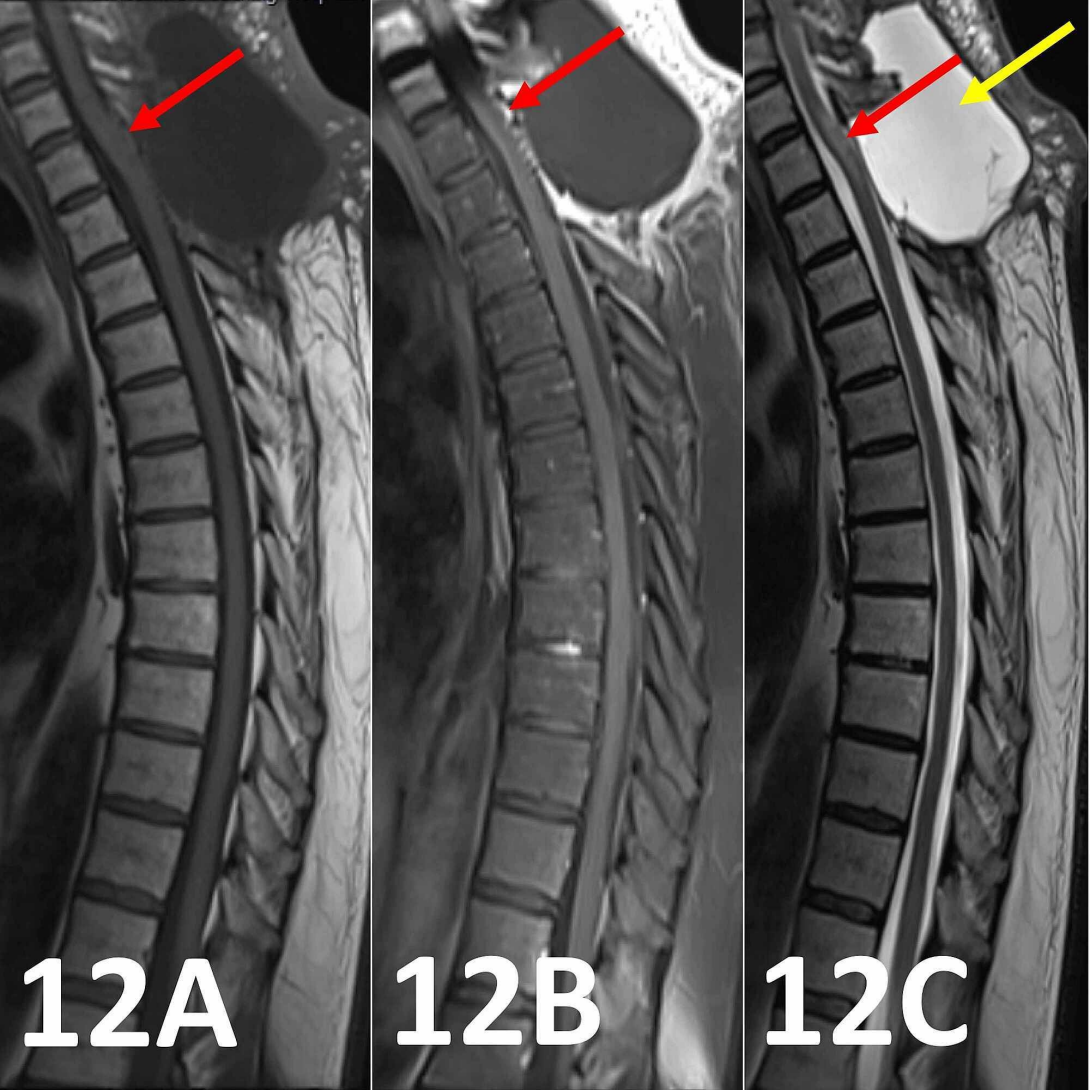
MRI of the thoracic spine with contrast 10 days post-operatively. (12A and 12B) Pre- and post-contrast sagittal sequences demonstrate equivocal punctate dots of remnant enhancement within the spinal cord at the resection site indicating near-complete resection of the enhancing tumoral component (red arrows). (12C) High T2 (and low T1 in Figures 12A, 12B) contained collection consistent with a post-operative pseudomeningocele abutting the posterior dura with few intrinsic septations (yellow arrow). The spinal cord is tethered to the posterior dura at the T1 vertebral level. Minor high T2 intrinsic spinal cord signal from the inferior endplate of C7 to the superior endplate of T2 has improved in comparison to preoperative MRI (red arrow).
